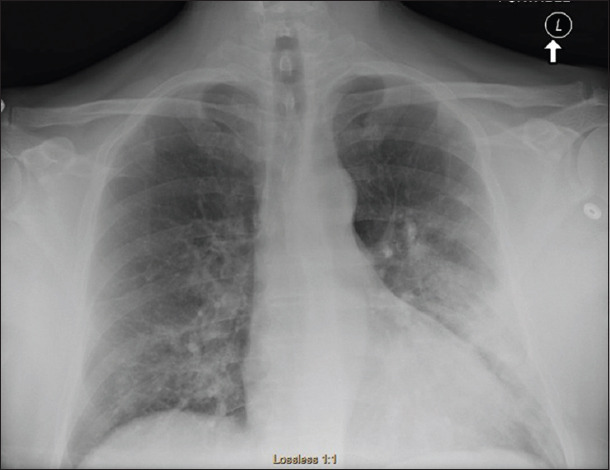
Chest radiograph showed that two frontal views of the chest are without comparison. Cardiac silhouette and pulmonary vasculature are normal. There is a left upper lobe infiltrate consistent with pneumonia. Epigastrium and skeletal structures are unremarkable.
